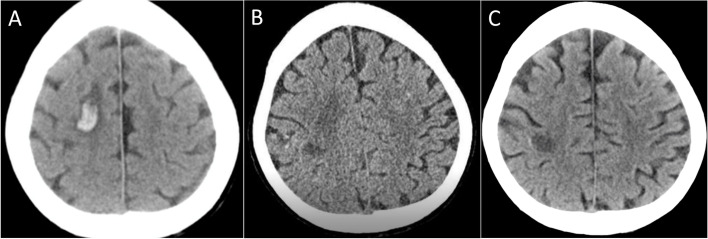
Changes in brain computed tomography (CT) findings. A On admission showing hemorrhagic cerebral infarction. B Before operation showing the regression of cerebral hemorrhage. C Before discharge showing no recurrence of cerebral hemorrhage
